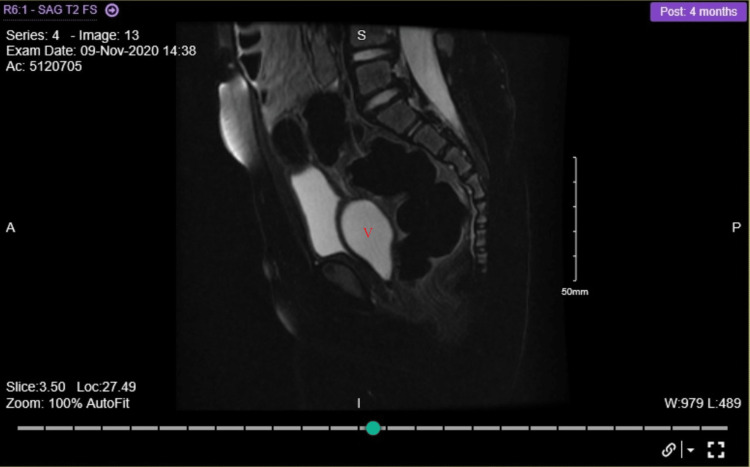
Magnetic resonance imaging of the pelvis demonstrating a fluid-filled vagina (red V)
